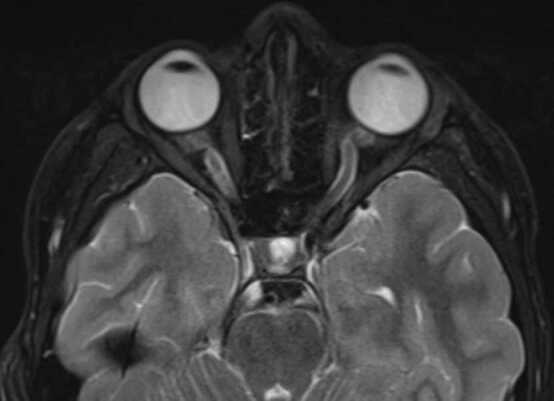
T2 MRI of the brain and orbits showing bilateral optic nerve dilation and enhancement suggestive of optic perineuritis.
